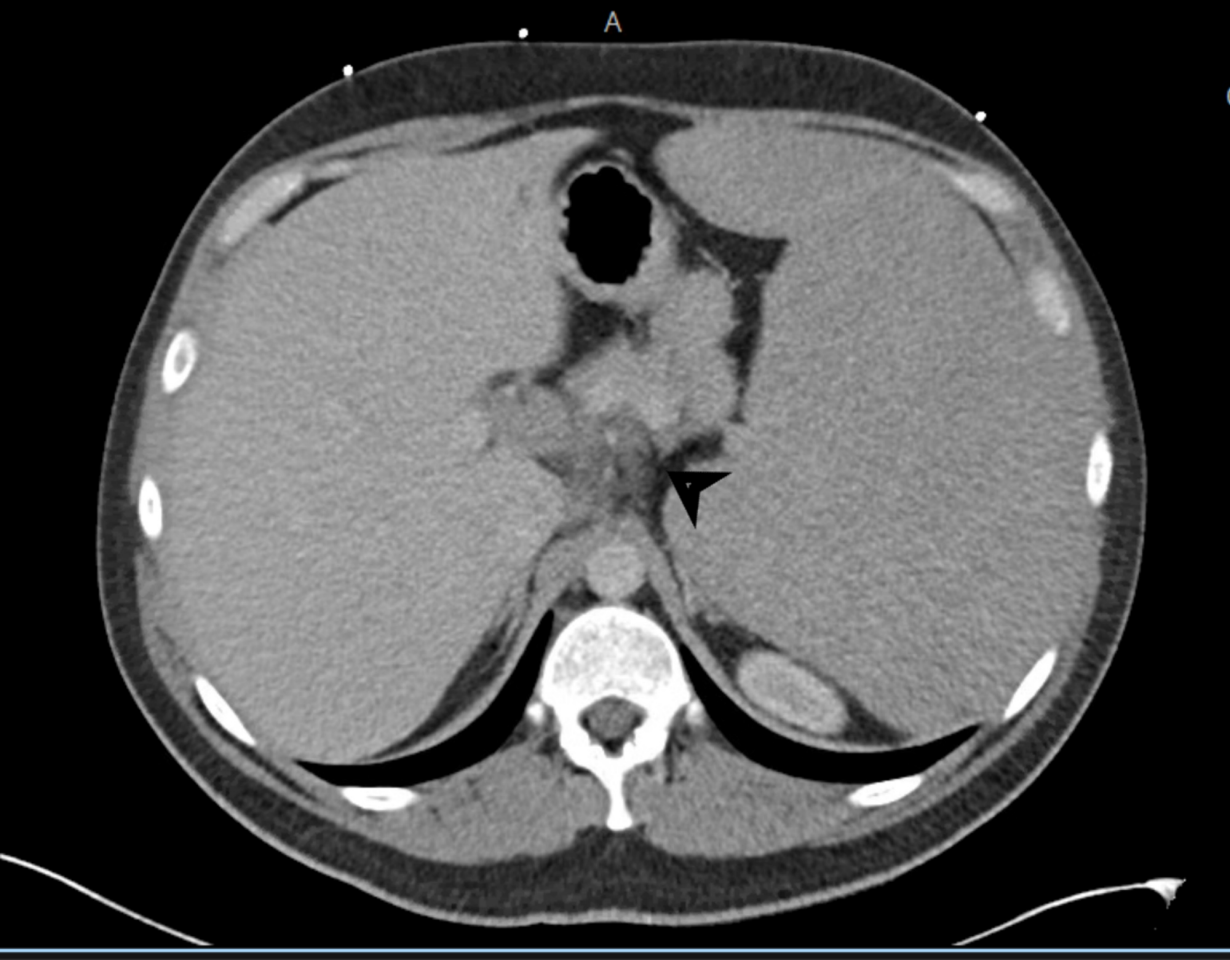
CT abdomen and pelvis with IV contrast demonstrating a 2.6 x 1.4 cm celiac axis lymph node.
